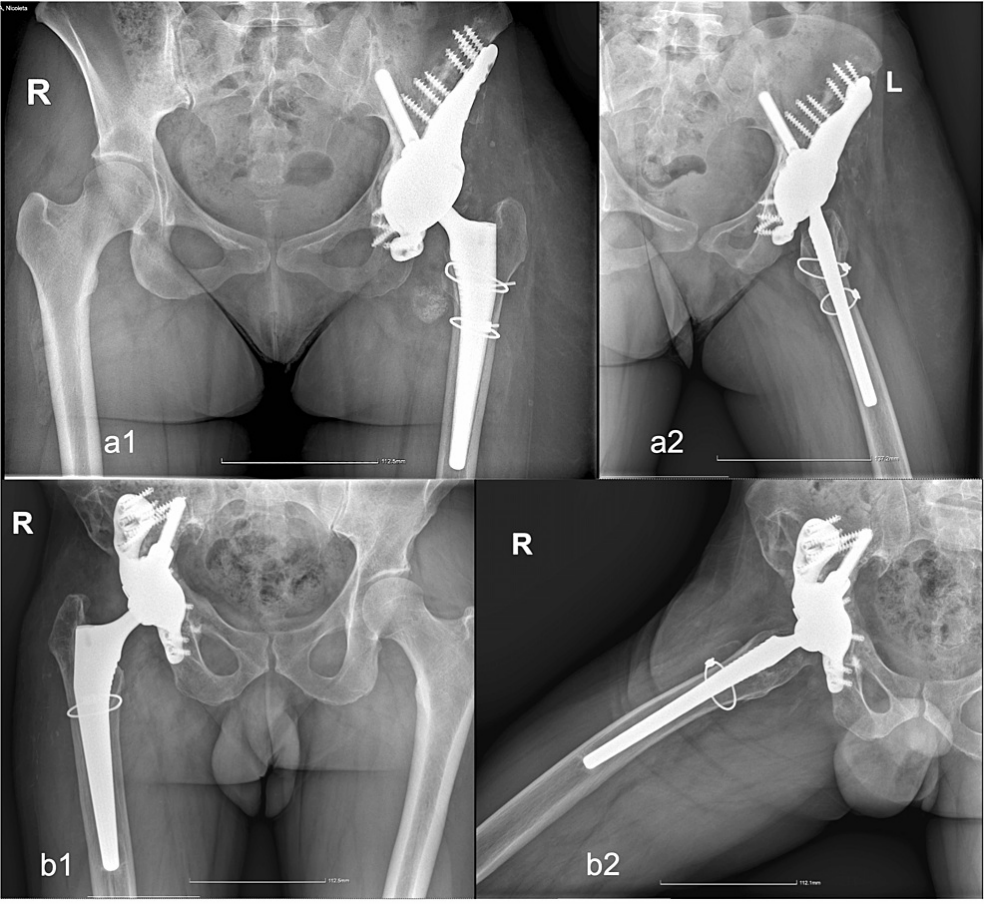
Radiographic control at follow-up Radiographic investigation at one-year follow-up (a: Case 1) and two years (b: Case 2).
